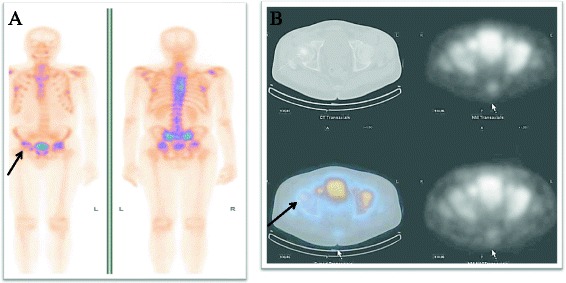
a Whole body bone scintigraphy 99mTc-MDP. Moderate uptake in the right shoulder, on both sides of the ribs due to osteoblastic lesions. Reduces radiotracer uptake in right femoral head (*long arrow*). b 99mTc-MDP SPECT/CT of the pelvis. Reduced radiotracer uptake in right femur head, corresponding sclerotic lesion on CT (*long arrow*) (year 2011)
